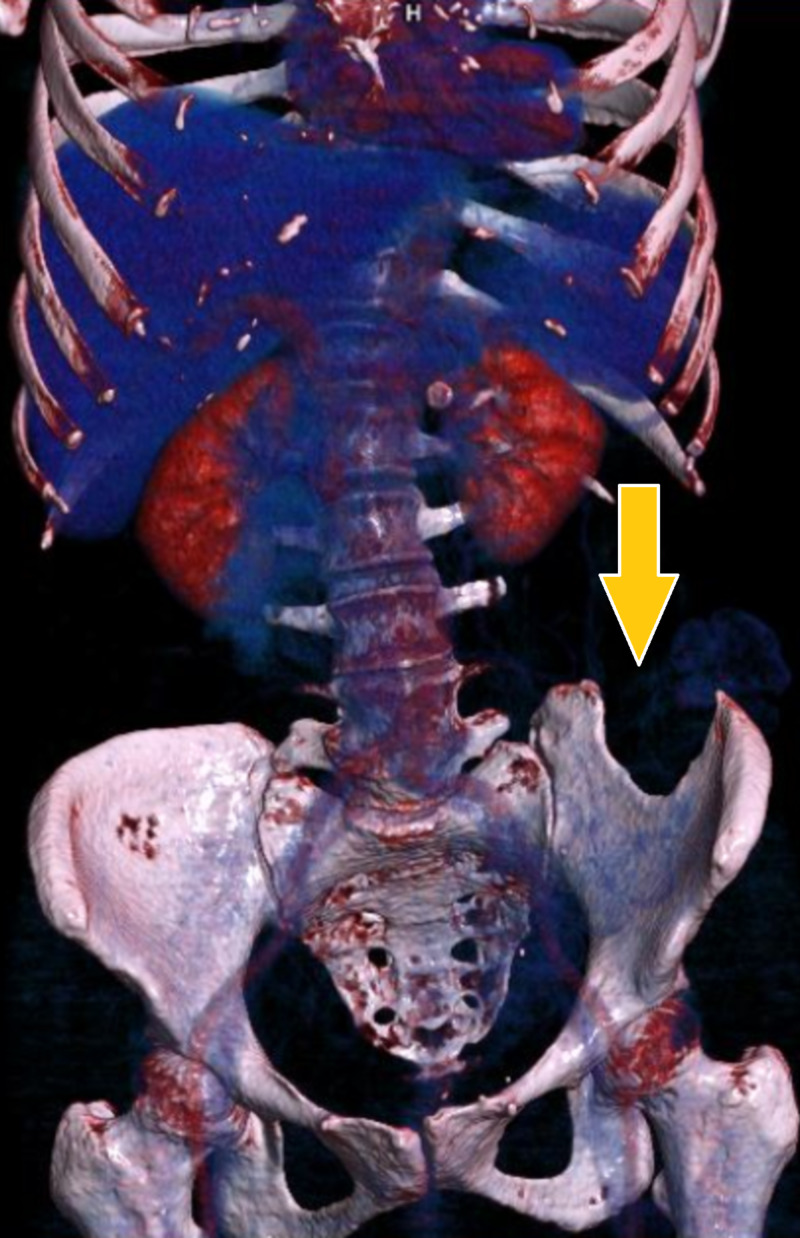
3D reconstructed contrast-enhanced CT image showing the defect in left iliac bone (arrow).
